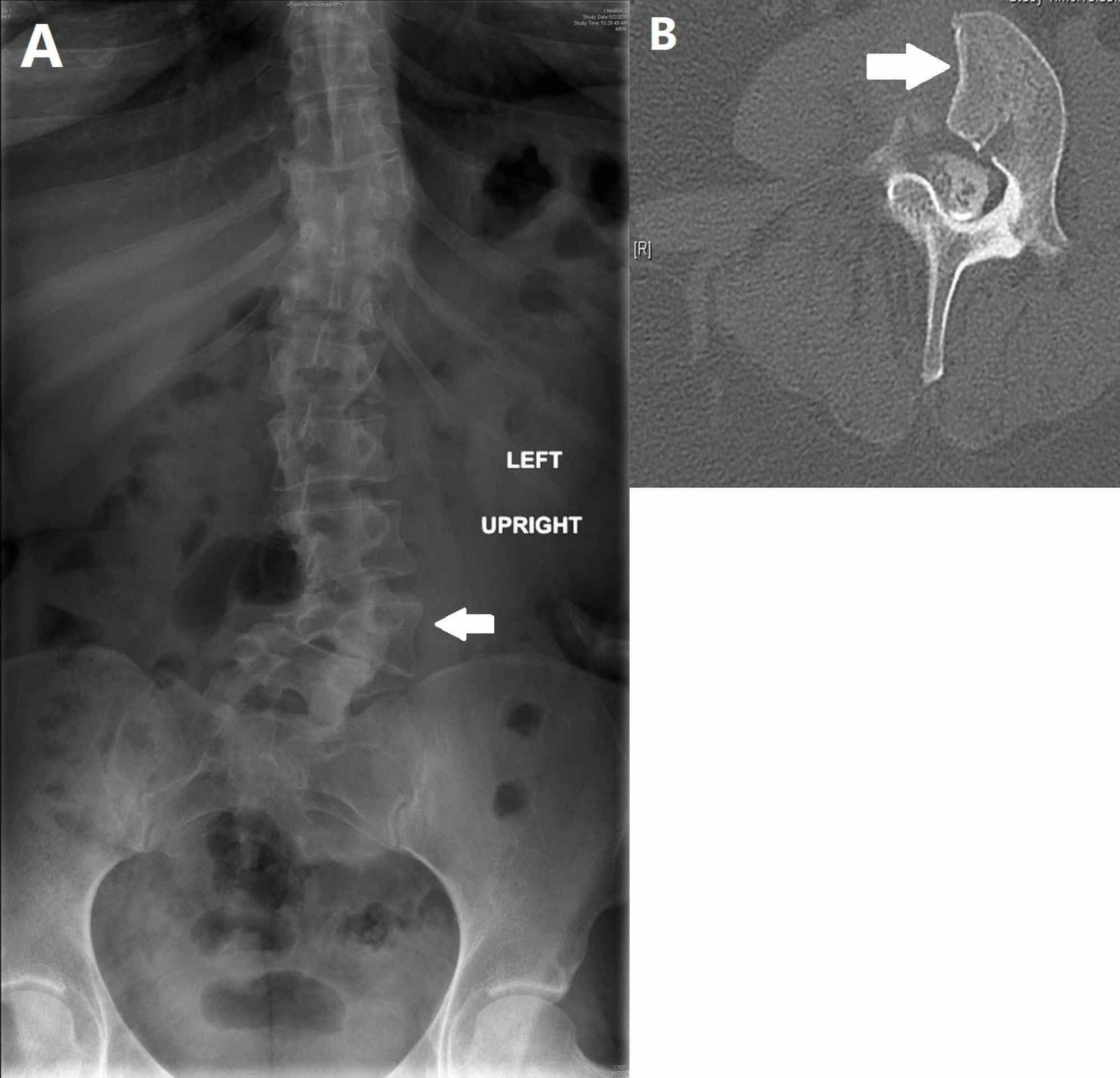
Lumbar hemivertebrae Anterior-posterior radiograph showing a lumbar hemivertebrae (arrow) and associated spinal curvature (A). An axial view of the same defect (arrow) on MR imaging (B)
